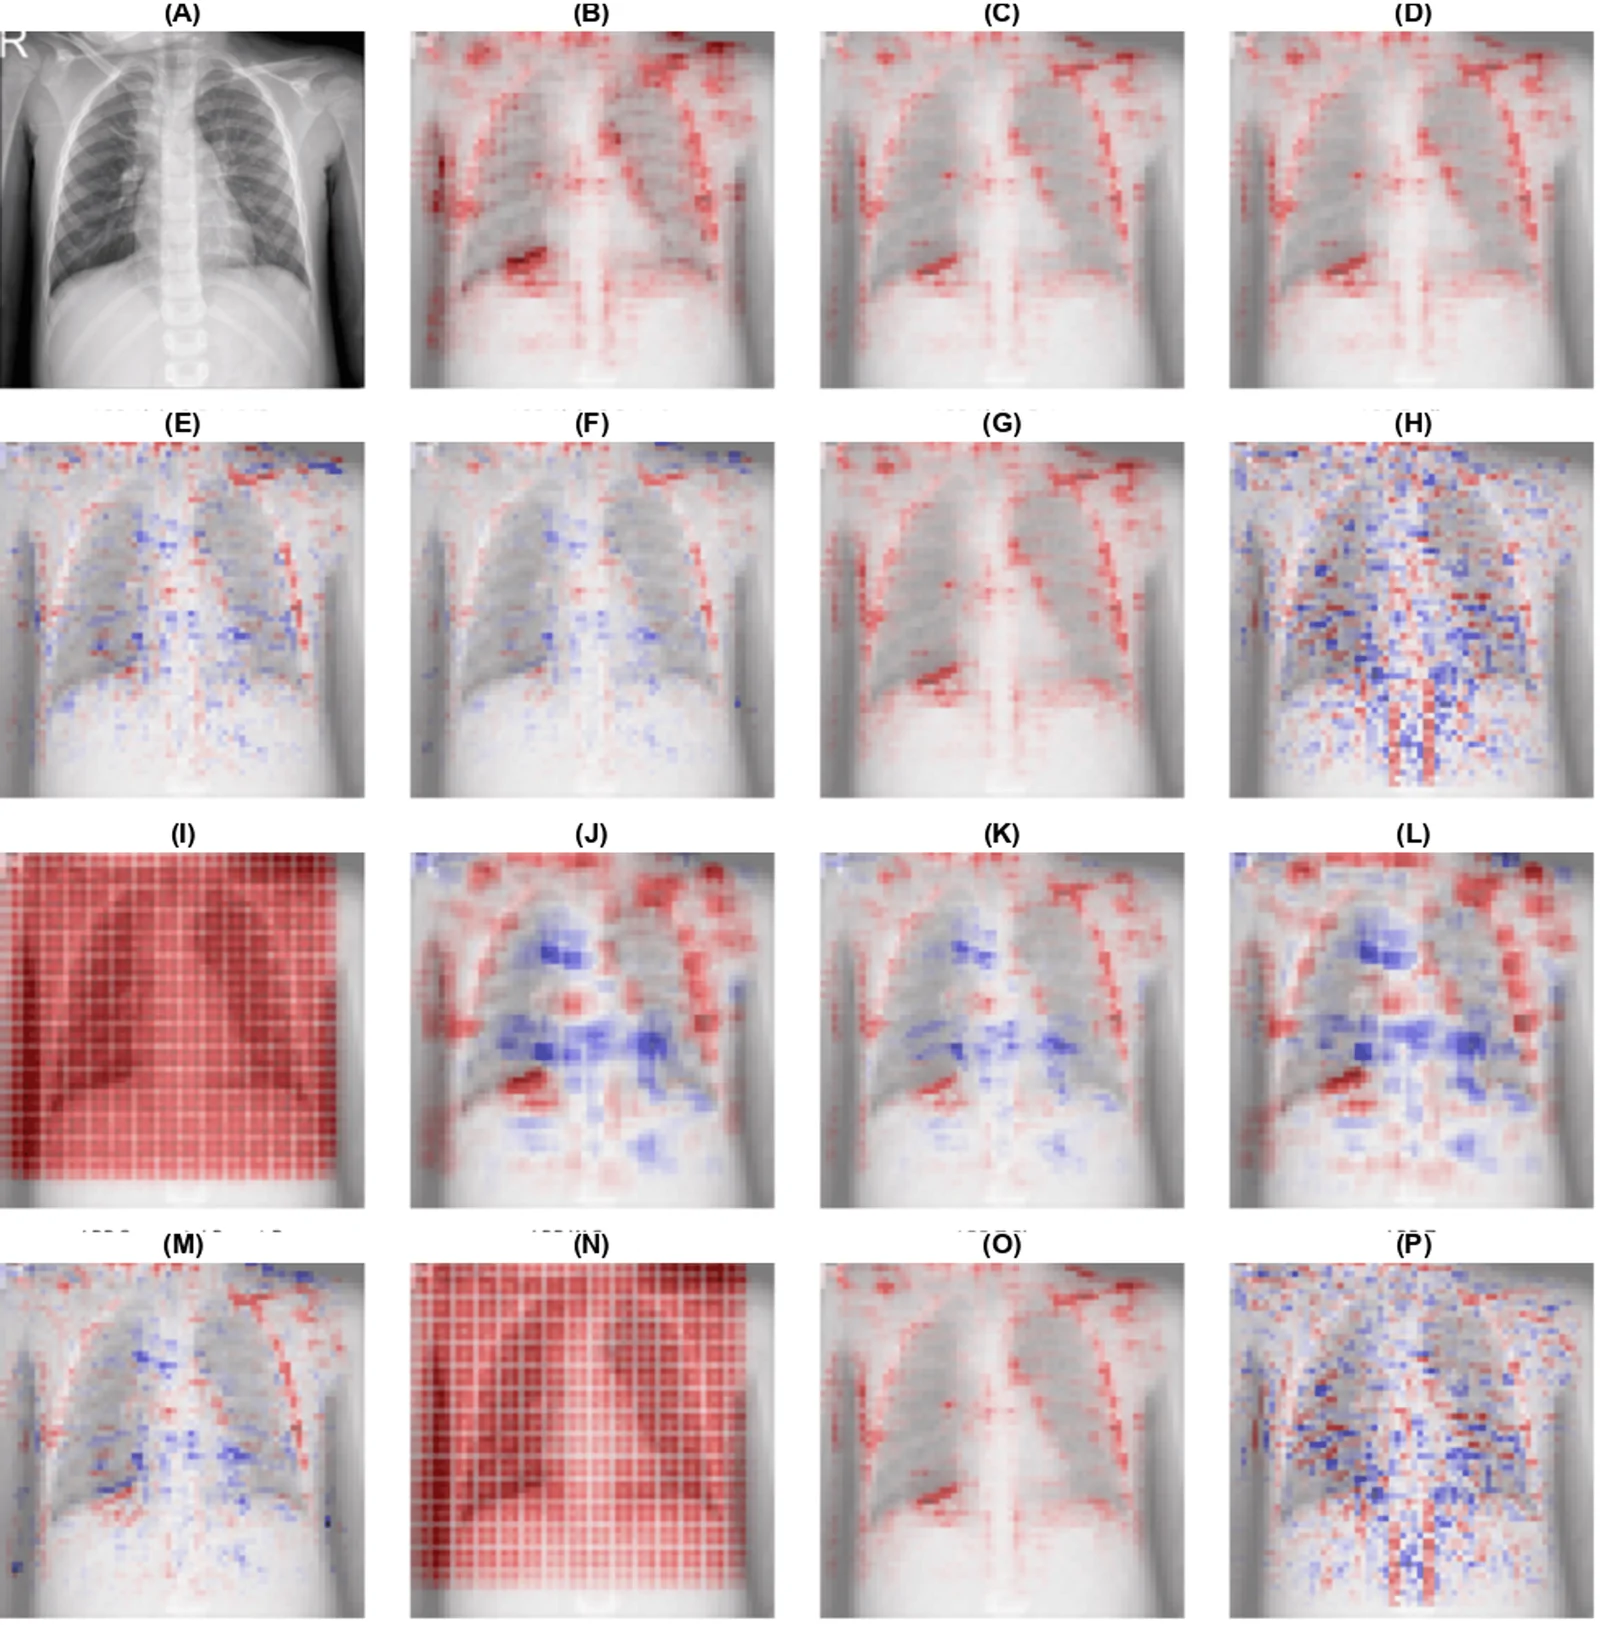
Representative pneumonia chest X-ray analyzed with Deep Taylor (bounded/unbounded) and LRP rules for the 2-class model (normal vs. pneumonia). Panels show the original image and corresponding relevance maps for each method. (A) Original chest X-ray; (B) Deep Taylor Bounded; (C) Deep Taylor; (D) LRP Alpha 1 Beta 0; (E) LRP Alpha 2 Beta 1 IB; (F) LRP Alpha 2 Beta 1; (G) LRP Alpha Beta; (H) LRP Epsilon; (I) LRP Flat; (J) LRP Sequential Preset A Flat; (K) LRP Sequential Preset A; (L) LRP Sequential Preset B Flat; (M) LRP Sequential Preset B; (N) LRP W Square; (O) LRP Z Plus; (P) LRP Z. LRP: layer-wise relevance propagation; IB: input-layer bounded Panel (A) was adapted from a case contributed by Yaïr Glick, Radiopaedia.org, rID 92316 [20], and is used under the Creative Commons Attribution-NonCommercial-ShareAlike 3.0 Unported license (CC BY-NC-SA 3.0); all relevance maps and annotations were generated by the authors.
